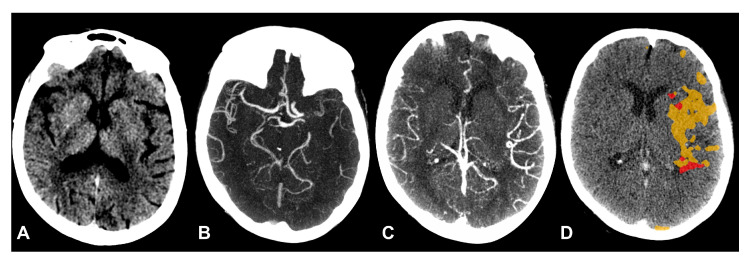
Patient with aphasia and right hemiparesis, last seen well two hours ago. There is subtle hypoattenuation on left lentiform nucleus (A). On CTA (B), there was poor filling of distal branches in MCA territory (sCTA 1). The venous phase extracted from CTP source-images (C) demonstrates good and delayed collateral filling (dCTA 2). The patient had a small core (red) and large penumbra (orange) volumes on CTP (D).
